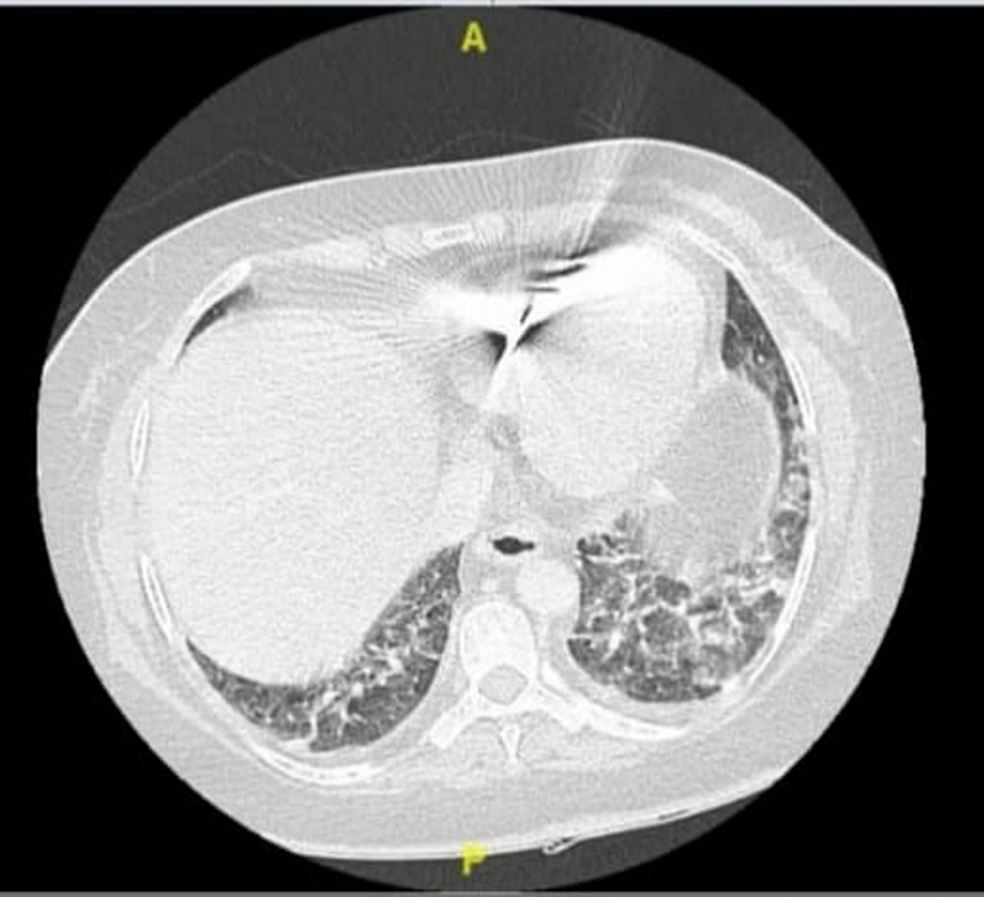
Computed tomography (CT) scan showing bilateral lower lung lobes patchy ground-glass opacification and atelectasis.
